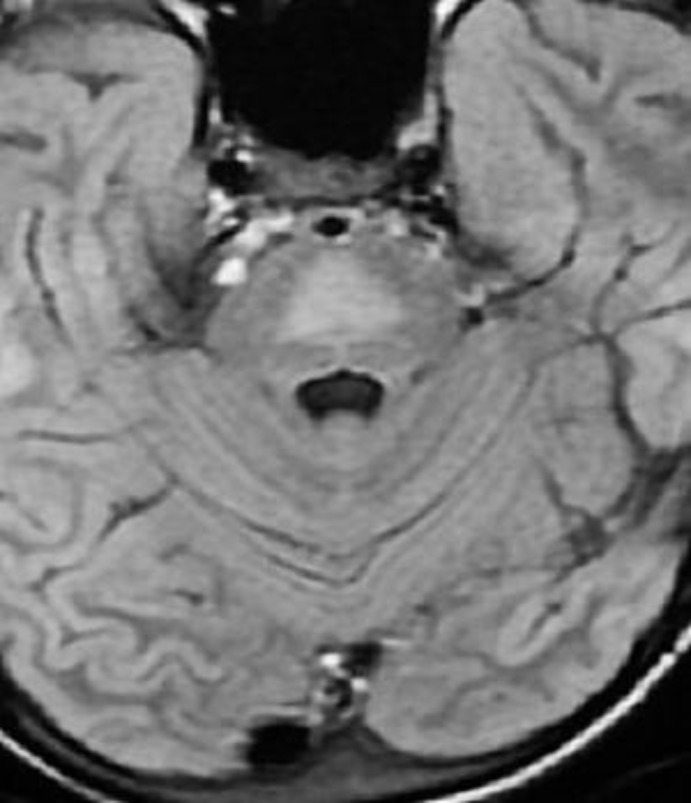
MRI brain, T2 Axial FLAIR showing trident shaped hyper-intensity in the central portion of pons.
